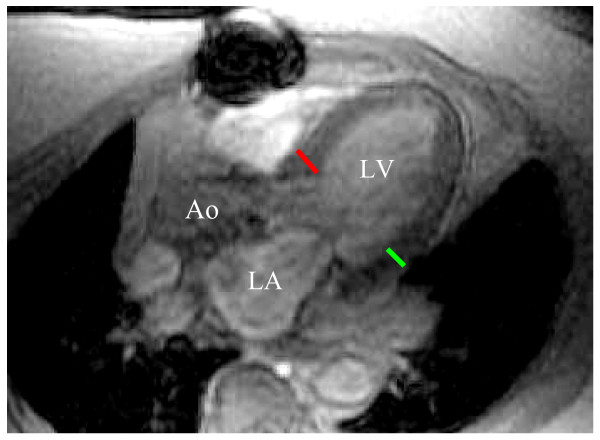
In accordance with the American Society of Echocardiography, posterior (green line) and septal (red line) left ventricular wall thickness was measured in the left ventricular long axis view at end diastole at approximately the level of the mitral valve leaflet tips (LV = left ventricle; LA = left atrium; and Ao = aorta).
